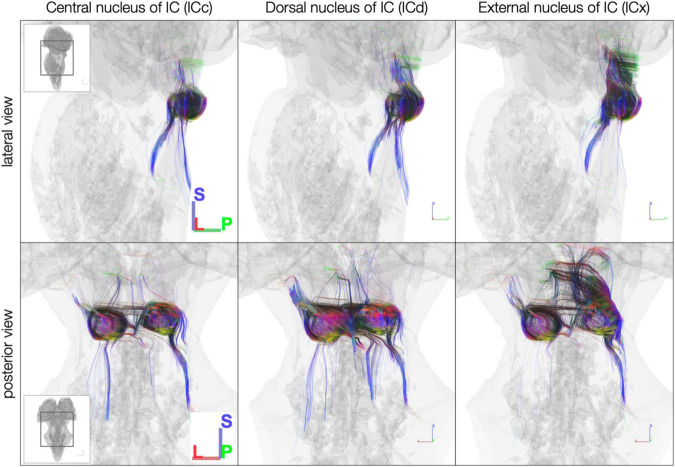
Diffusion MRI tractography streamlines reaching each of the inferior colliculus subdivisions in the *post mortem* dataset. Colors represent local streamline orientation: blue, superior–inferior; red, left–right; green, anterior–posterior.
